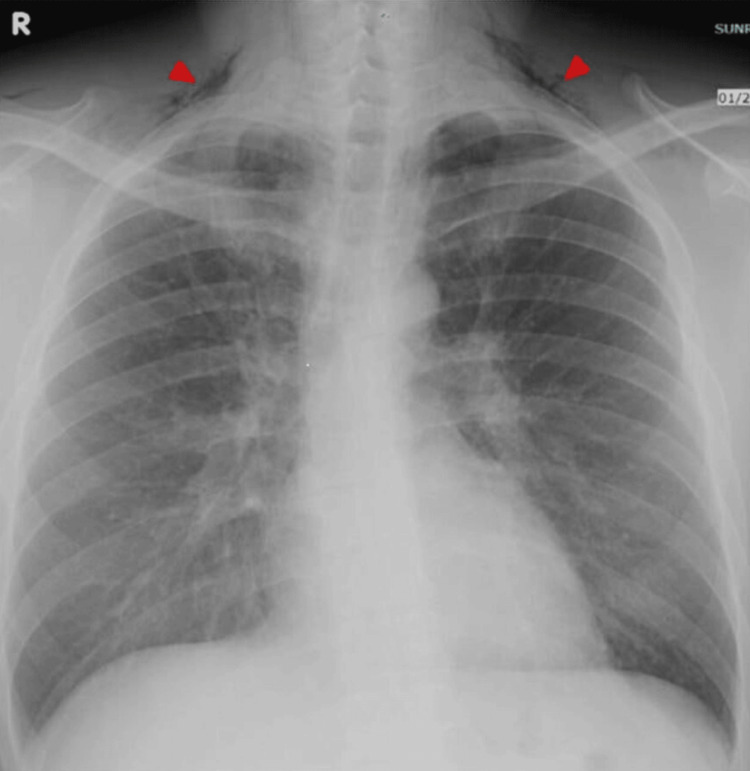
Chest X-ray revealed free air at the cervicothoracic junction (red arrows).
